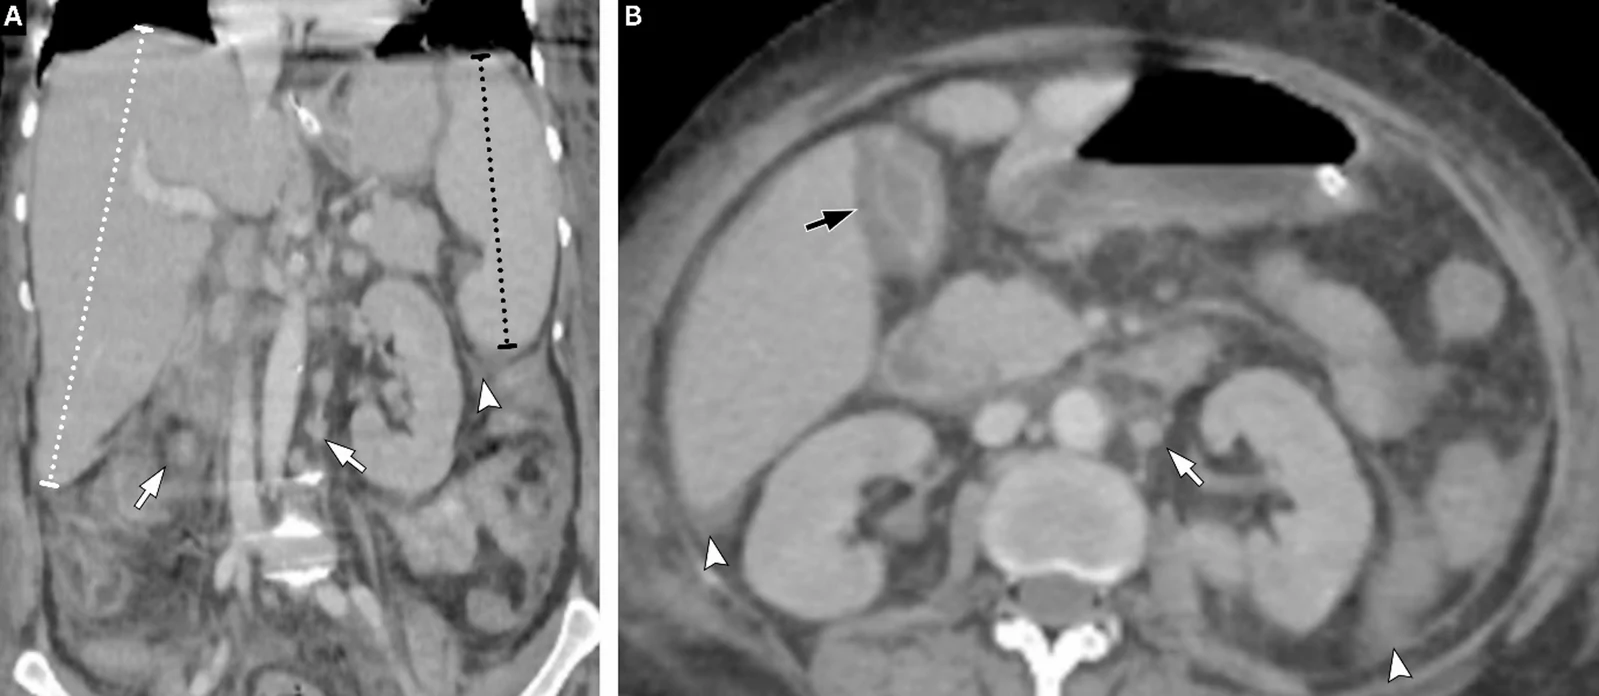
Secondary hemophagocytic lymphohistiocytosis (HLH) due to scrub typhus in a 58-year-old male. Coronal (A) and axial (B) contrast-enhanced CT images demonstrate hepatomegaly (white dotted line), splenomegaly (black dotted line), mesenteric and para-aortic lymphadenopathy (solid white arrows), gallbladder wall edema (solid black arrow), with trace ascites (white arrowheads). Laboratory workup showed elevated liver enzymes, neutropenia, thrombocytopenia, hyperferritinemia, and hypofibrinogenemia fulfilling criteria for secondary HLH
